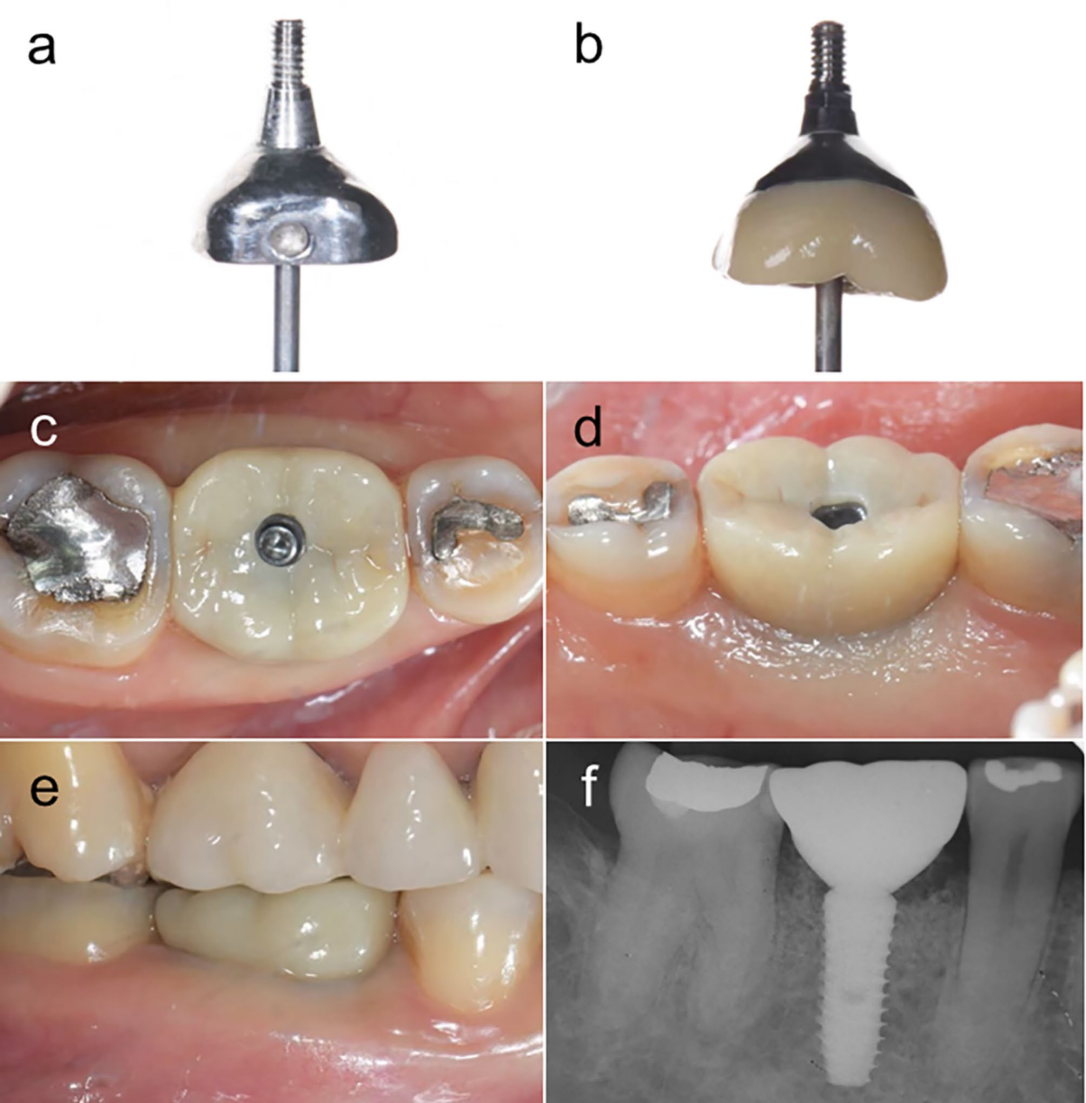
(a), (b) The outline form comparison between the customized titanium healing abutment and final prosthetic abutment with zirconia crown, (c) occlusal view, (d) lingual view, (e) buccal view, (f) radiographic examination: The final screw-retained implant prosthesis was complete after 6 months
